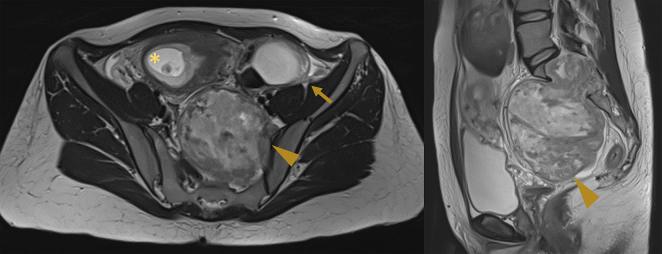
No-contrast magnetic resonance imaging during pregnancy. On the left, an axial scan showing the viable fetus (*), the multilocular solid left ovarian mass (arrows) and the retroperitoneal Schwannoma (arrowhead); on the right, a sagittal scan with the retroperitoneal Schwannoma (arrowhead).
